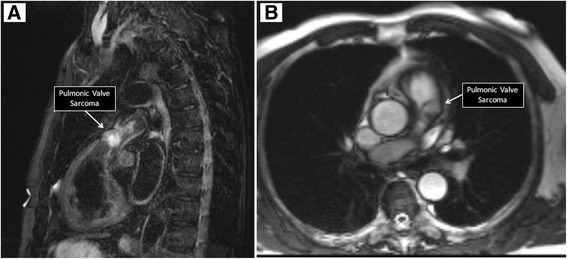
MRI. T2 weighted sagittal image showing spindle-cell sarcoma (Panel a). Still image from a TruFisp cine sequence, showing the location of the mass in the pulmonary artery (Panel b)
